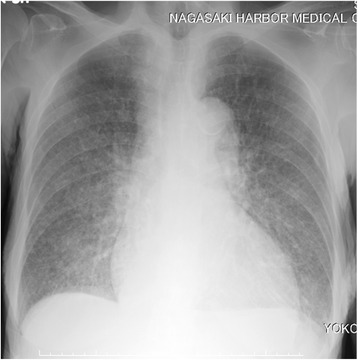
Chest radiography on admission showed diffuse micronodules in both lung field
